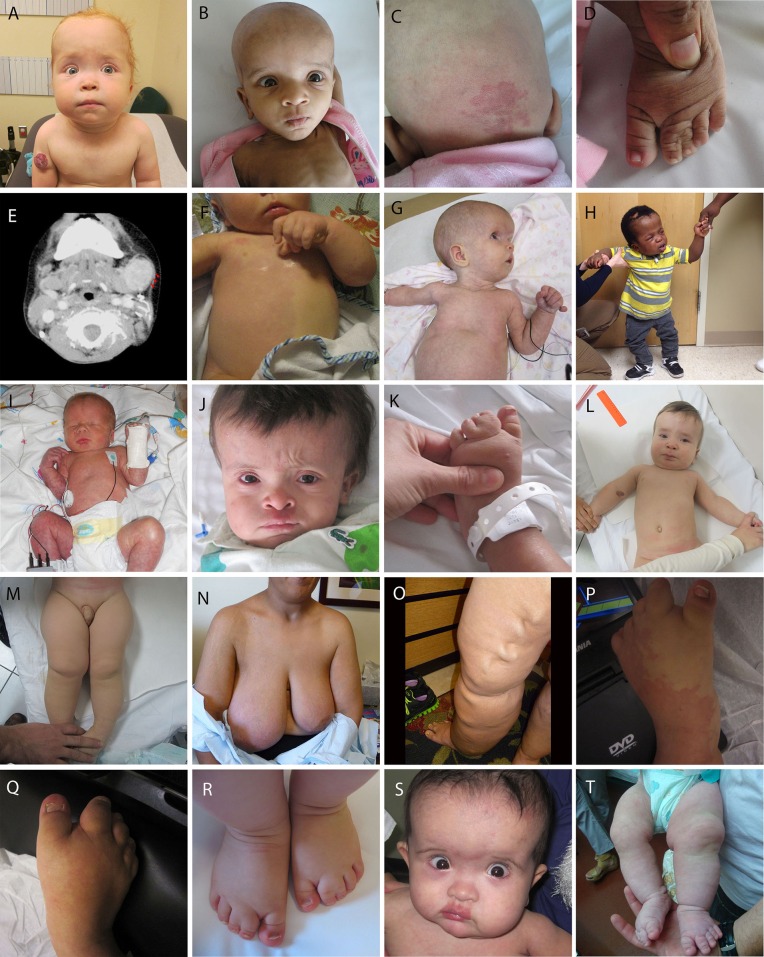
Clinical photographs of MCAP patients. (A) Facial photograph of patient LR14-323 (p.Arg83Gln). (B–D) Photograph of the face (B), occipital region (C), and left foot (D) of LR13-359 (p.Pro104Leu) showing MEG, occipital capillary malformation, and syndactyly of the second, third, and fourth toes. (E) Computed tomography (CT) image of LR01-060 (p.Pro104Leu) showing the subcutaneous hemangioma (arrowheads). (F) Photograph of the trunk of LR12-080 (p.Arg115Pro) showing cutaneous capillary malformation with midline delineation. (G) Photograph of LR12-365 (p.Asn345Thr) showing diffuse capillary malformations, MEG with a prominent forehead, and postaxial polydactyly of the left hand. (H) Photograph of LR13-036 (p.Glu365Lys) showing MEG, a disproportionately small body, and short extremities (clinically diagnosed with rhizomelic shortening of the extremities). (I) Photograph of LR11-418 (p.Cys378Tyr) showing diffuse capillary malformations and apparent megalencephaly (MEG). (J and K) Photograph of the face (J) and left foot (K) of LR12-330 (p.Glu545Asp) showing clear MEG, capillary malformation of the philtrum, skin laxity of the forehead, and syndactyly of the second, third, and fourth toes. (L and M) Photographs of the face (L) and body (M) of LR13-038 (p.Gly914Arg) showing MEG, reticulated capillary malformations, pigmented nevus of the right arm, and asymmetry of the legs. (N and O) Photographs of the chest (N) and lower extremity (O) of LR12-383 (p.Gly914Arg) showing clear asymmetry of the trunk involving the right breast and overgrowth of the right leg with prominent venous network. (P and Q) photographs of the left (P) and right (Q) feet of LR11-081 (p.Thr1025Ala) showing bilateral asymmetric macrodactyly, sandal-gap toes, and capillary malformations. (R) Photograph of the feet of LR13-169 (p.Ala1035Thr) showing syndactyly of the second, third, and fourth toes on the right, and second and third toes on the left. (S and T) photographs of the face (S) and lower extremities (T) of LR12-328 (p.Met1043Ile) showing facial and body asymmetry, MEG with a prominent forehead, and capillary malformations on the face and body.
